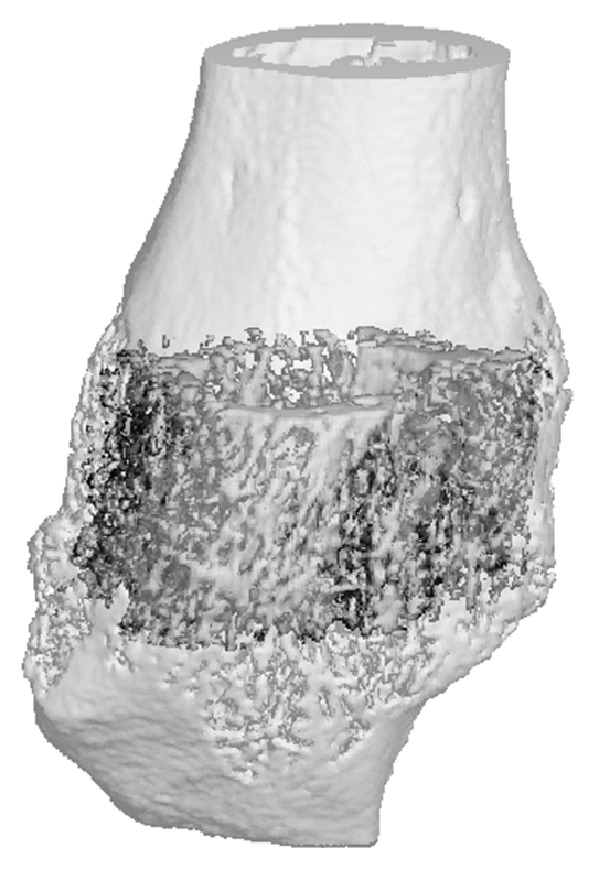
Hybrid *μ*-CT image of the fracture region. The callous region is colored darker.
